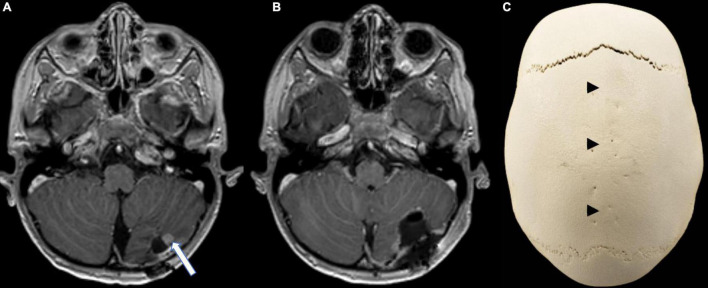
Follow-up imaging. (A) Axial contrast-enhanced T1-weighted MR image showing a contrast enhancing nodule (arrow) at the infero-lateral border of the resection cavity, strongly suggestive of local recurrence. (B) Axial contrast-enhanced T1-weighted MR image after en-bloc resection of the recurrent tumor. (C) CT reconstruction (Volume rendering) of the skull highlighting the dolichocephaly and the premature synostosis of the sagittal suture (arrow heads) when compared to the open sutures.
